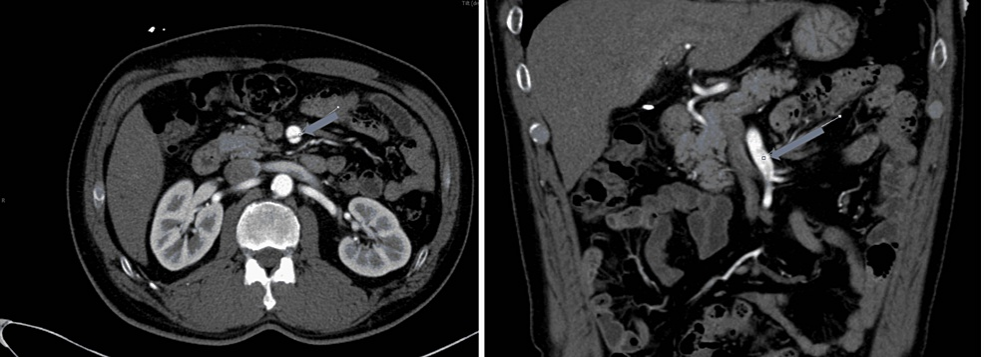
CT angiogram of abdomen and pelvis showing an intimal tear of the SMA SMA: superior mesenteric artery
